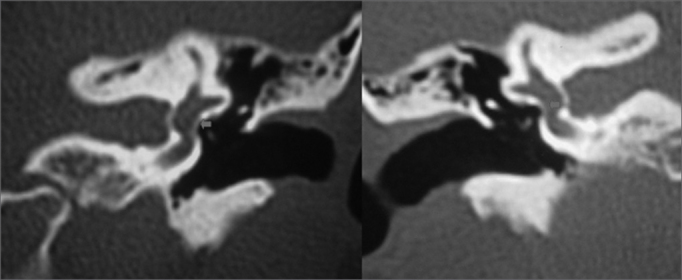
CT of the temporal bones in coronal slices along the oval window showing thickening of the stapes footplate (red arrows).
